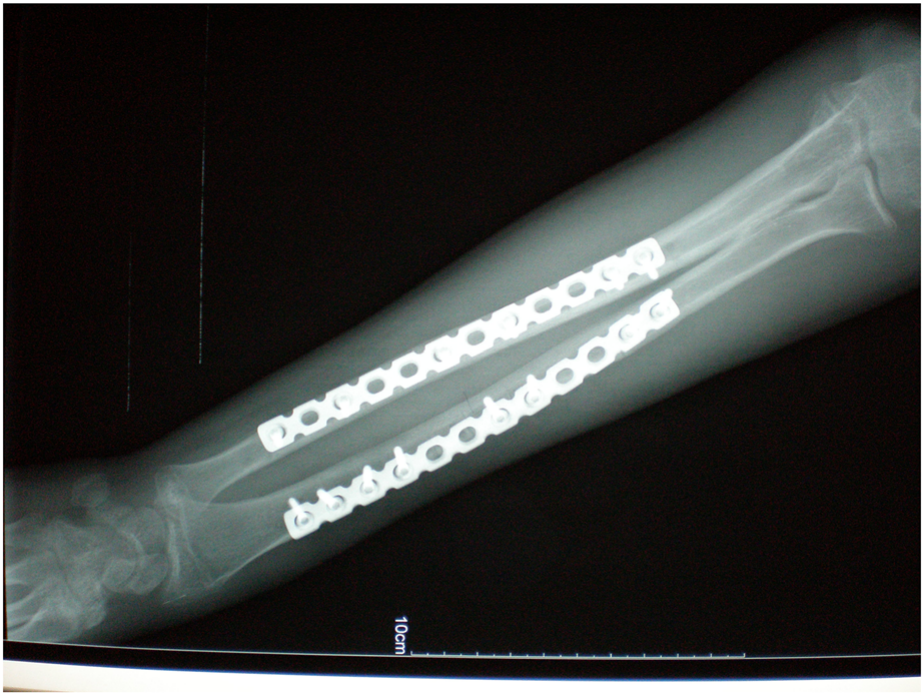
X-ray showing perfect anatomic reduction and healed insufficiency fractures caused by the multiple-level osteotomies.
